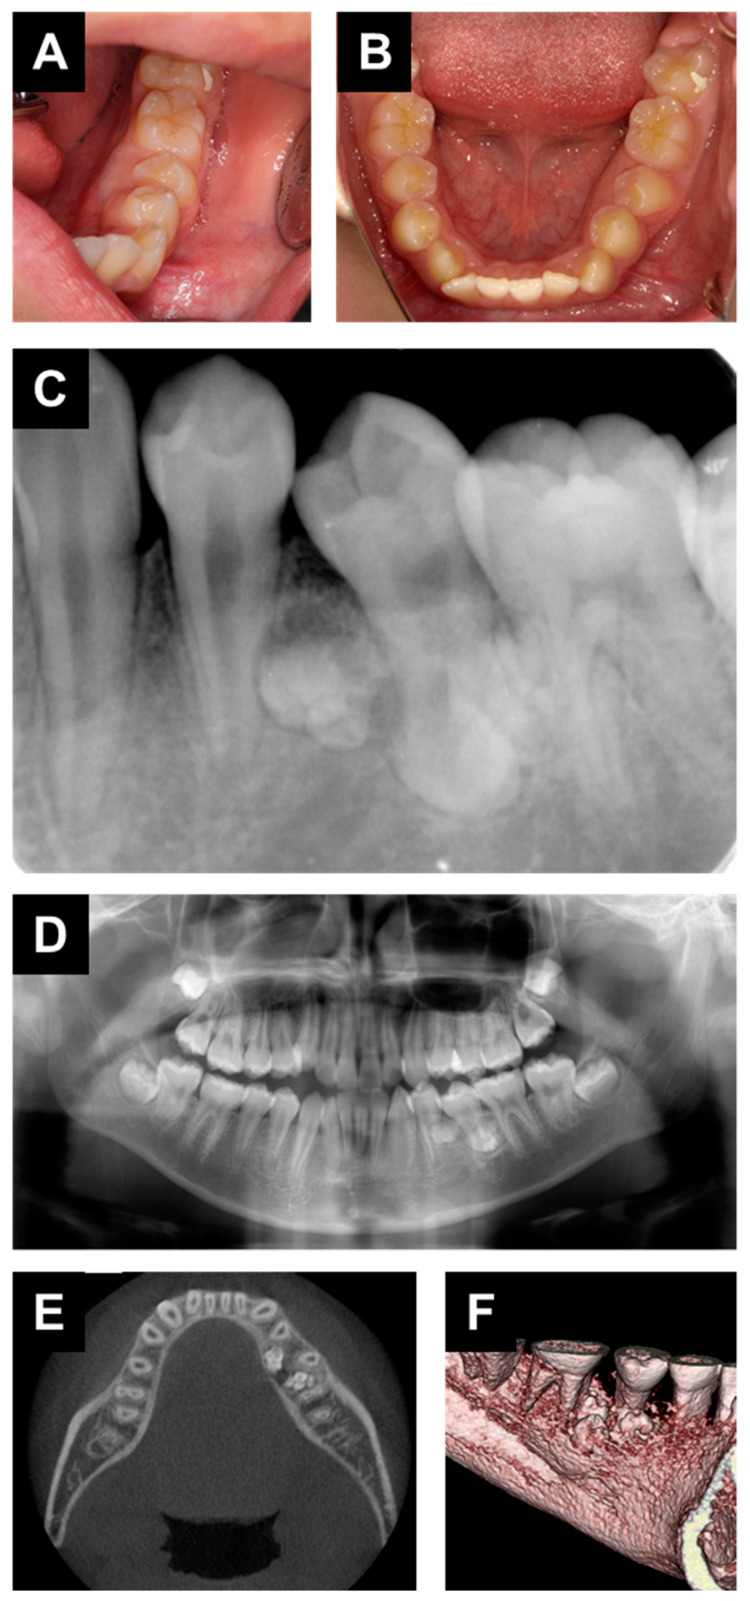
(A,B) Intraoral photographs taken at the initial visit. (C) Panoramic radiography, (D) periapical radiography, and (E) computed tomography images. (F) Three-dimensional construction of affected region.
